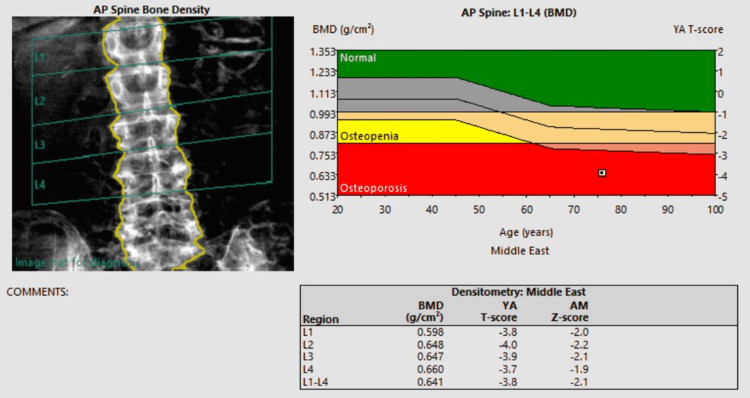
DEXA scan of the lumbar spine This image is the report of the patient's DEXA scan which shows her lumbar spine being in the osteoporotic range (T-score less than -2.5). DEXA: dual-energy X-ray absorptiometry; BMD: bone mineral density
